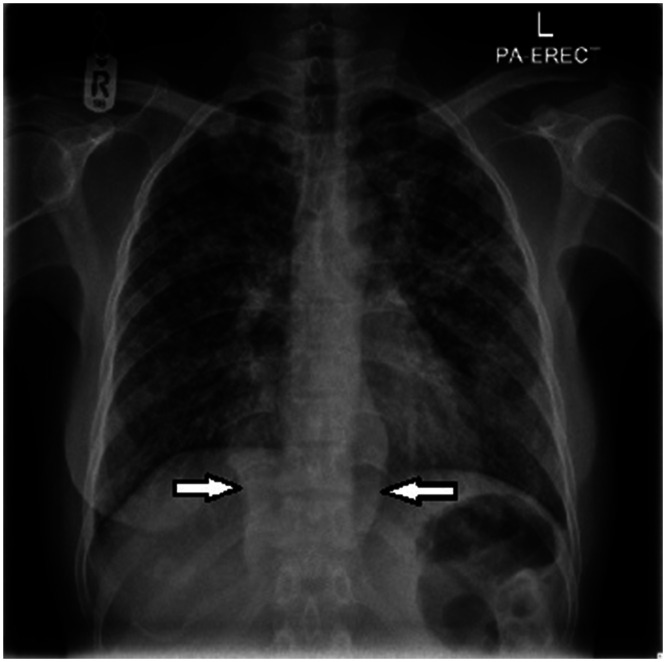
A chest X-ray showing extensive bilateral discrete and confluent nodular opacities in bilateral lungs. A bilateral paraspinal shadow is seen in the lower thoracic spine (arrows).
